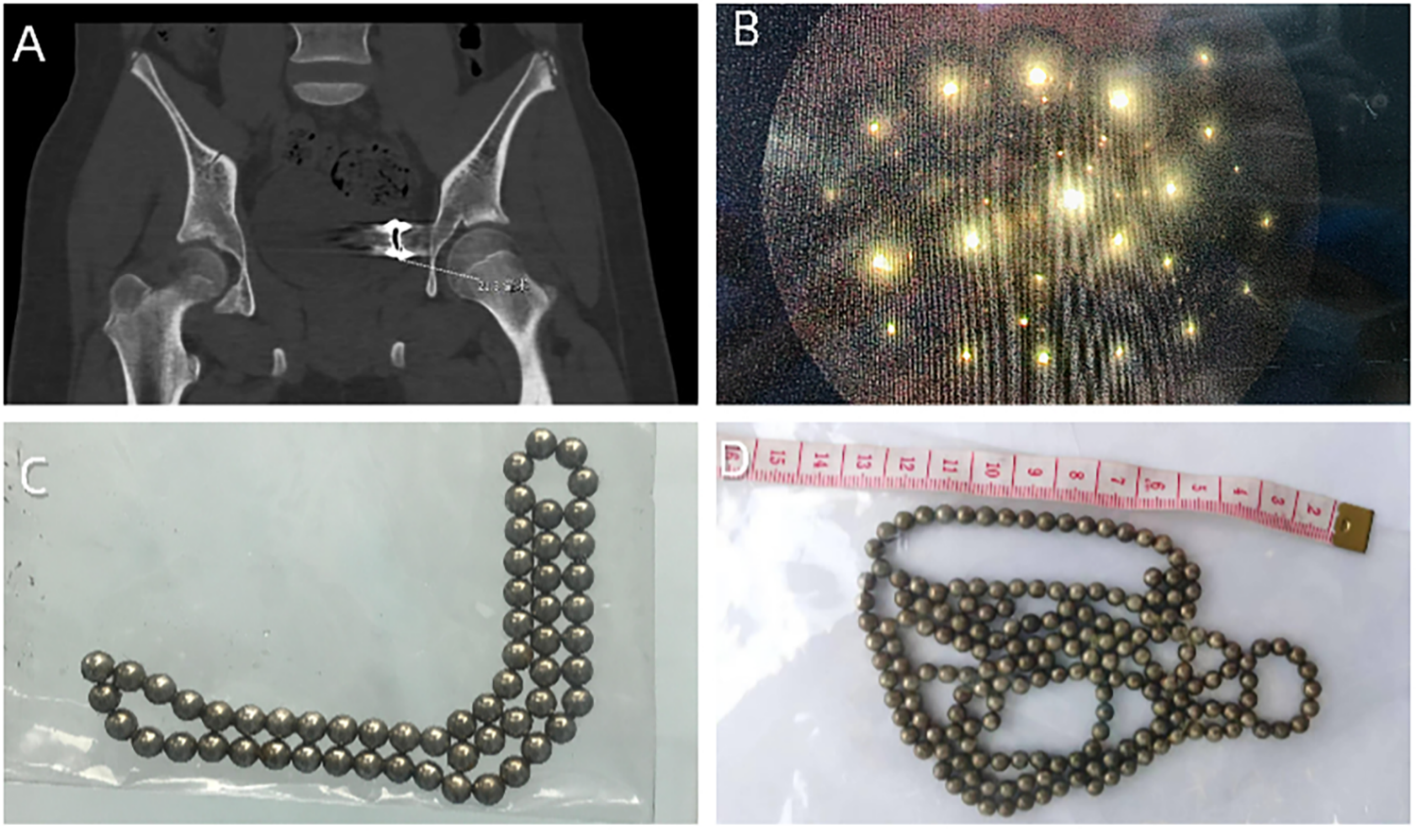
(A) A pelvic computed tomography (CT) scan showed a ring-shaped, bead-like high-density shadow closely adjacent to the left side of the bladder, considered to be a foreign body. (B) Cystoscopy revealed multiple magnetic beads in the bladder. (C) 59 magnetic beads of 5 mm in diameter. (D) 185 magnetic beads of 3 mm in diameter.
